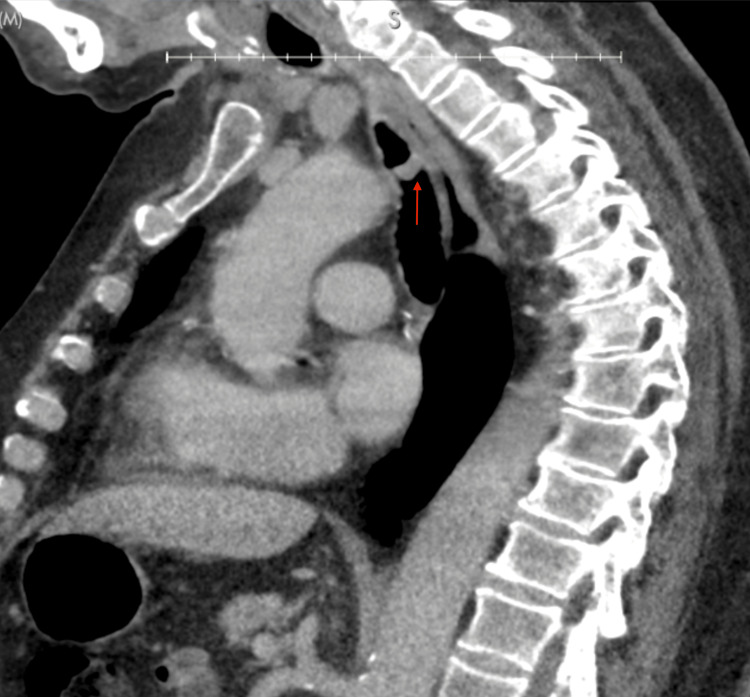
Sagittal view of the patient's noncontrast CT chest imaging. A metastatic tracheal lesion (red arrow) is demonstrated. The left mainstem bronchus lesion was not detected CT: computed tomography
